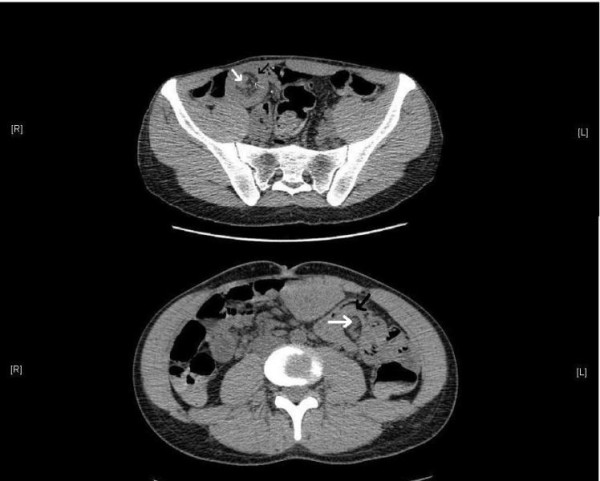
Axial computed tomography (CT) scan images demonstrating intussusceptions at different levels, with intussuscipiens (*single black arrow*), intussusceptum (*thick white arrow*) and vessels in the invaginated mesenteric fat (*thin white arrow*).
